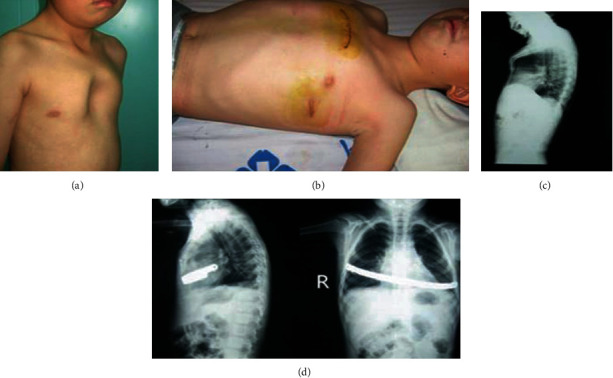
Pectus carinatum (pouter pigeon breast) comorbid with pectus excavatum: (a) preoperative appearance, (b) postoperative appearance and surgical incisions, (c) preoperative lateral chest X-ray film, and (d) postoperative anterior and lateral chest X-ray films.
